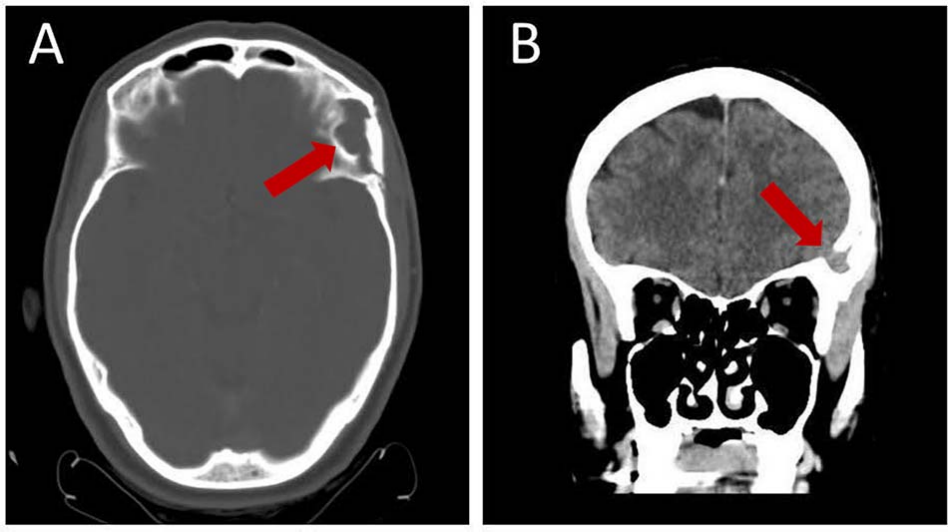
Follow-up brain CT, 3 months post-operation, showing complete resection of the lesion without residual tissue: (A) axial view and (B) coronal view.
